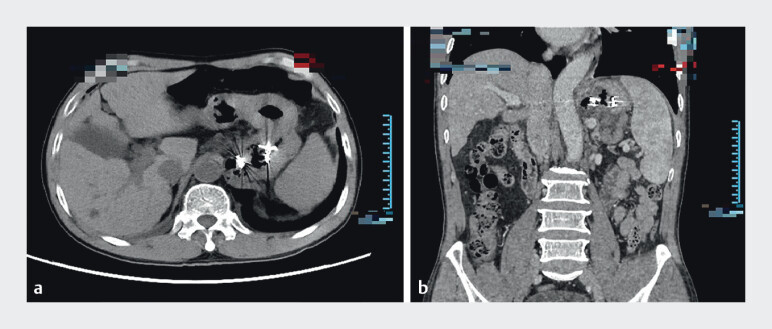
Follow-up abdominal and pelvic CTA after treatment showed the disappearance of varices and extramural vessels, with the GRS still present.
